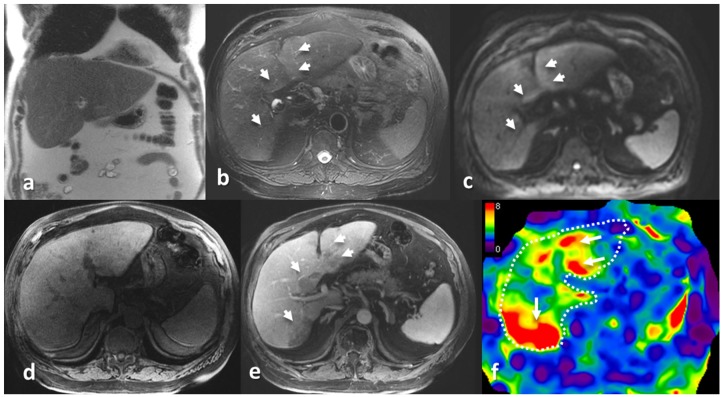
A 64-year-old male with known pulmonary amyloidosis on follow up. A follow-up CT (not shown) revealed hypodense lesions in the liver. An MRI with MRE was performed. The coronal T2-weighted image (a) shows borderline hepatomegaly (15.5 cm). The spleen was also borderline enlarged (12 cm). Multifocal lesions (arrow heads) were found in both lobes of the liver, which appeared hyperintense on the axial T2-weighted image (b) and diffusion weighted image (c), hypointense on the T1-weighted image (d), and hypoenhancing on the post contrast image (e). The MRE stiffness map (f) with a scale of 0–8 kPa shows moderately elevated liver stiffness (mean ± SD, 4.6 ± 0.8 kPa) with focal increased stiffness regions corresponding to the focal lesions. A percutaneous biopsy performed on the right lobe lesions confirmed hepatic amyloidosis.
